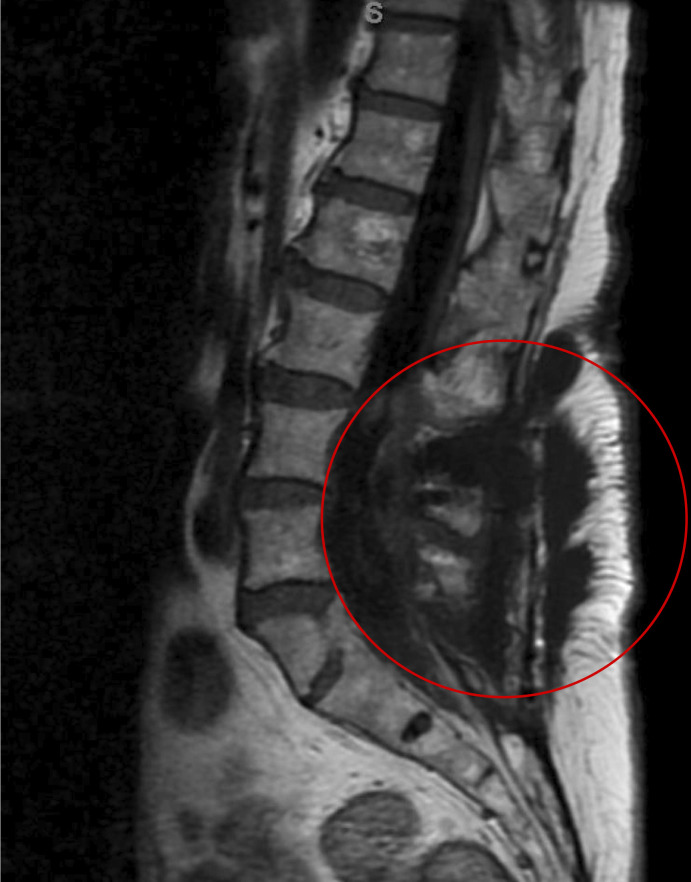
Magnetic resonance imaging of the lumbar spine on the day of admission. The red circle highlights the region of epidural fluid buildup.
